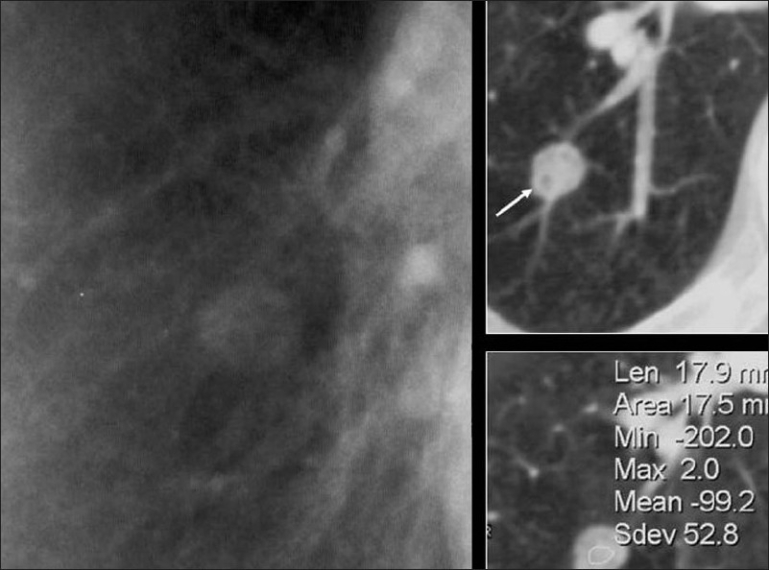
A blown-up image from a chest radiograph and axial CT scans showing low attenuation areas (arrow) within the PN due to fat virtually diagnostic of a hamartoma
